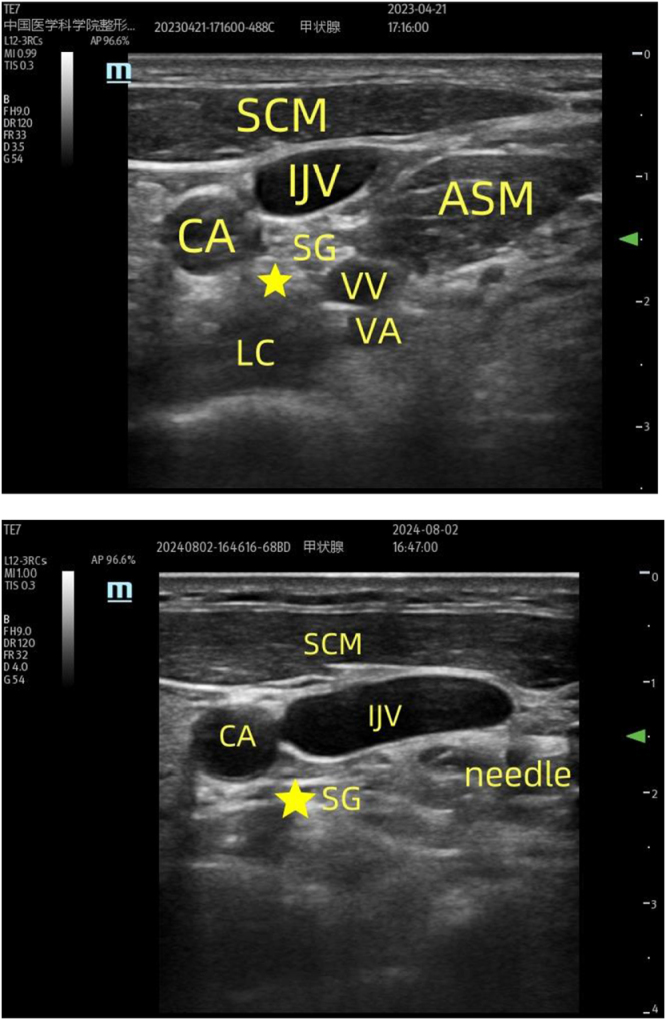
Ultrasound-guided stellate ganglion block. SG, stellate ganglion; SCM, sternocleidomastoid; ASM, anterior scalenus muscle; CA, carotid artery; IJV, internal jugular vein; VV, vertebral vein; VA, vertebral artery; LC, longus colli.
